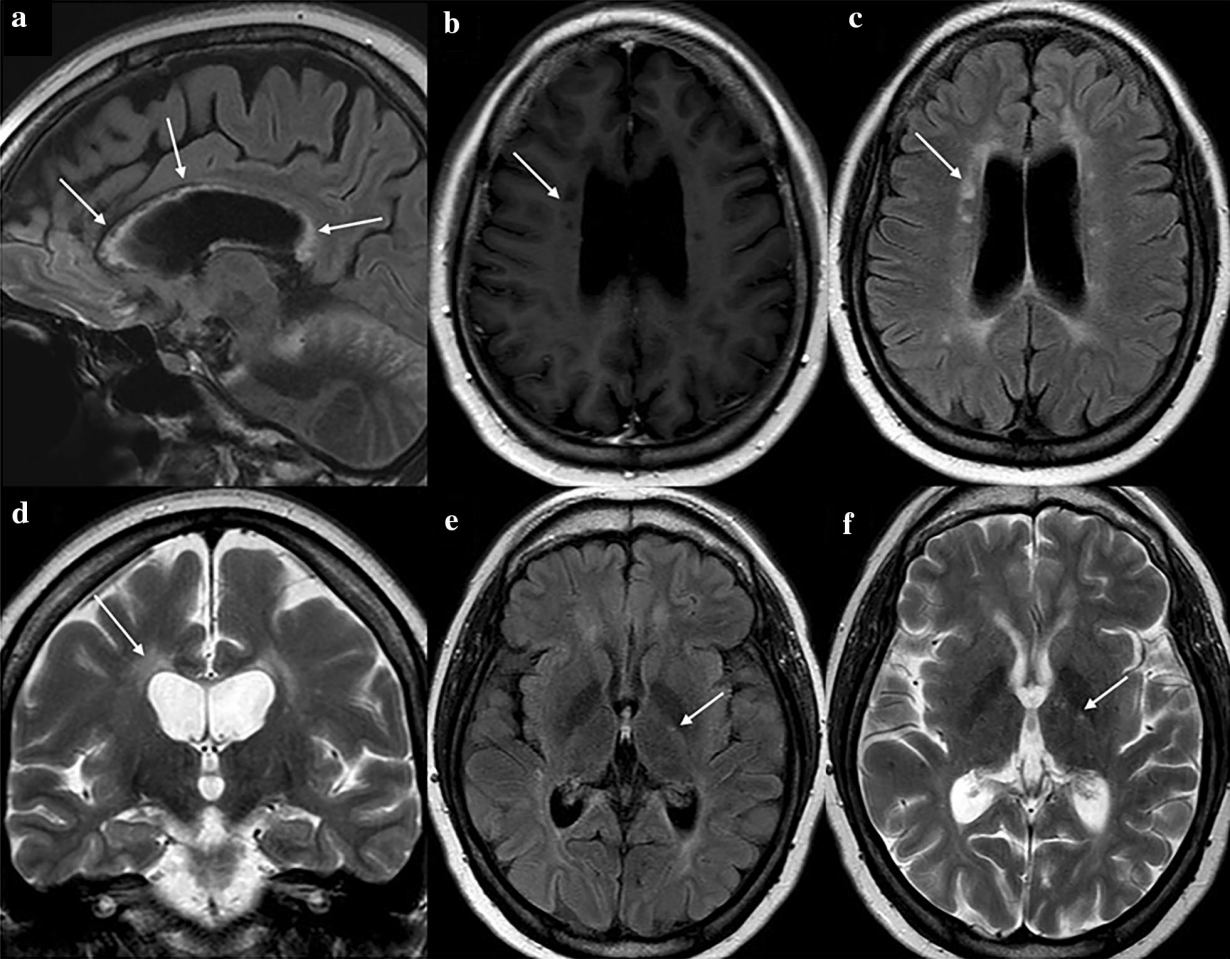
Brain magnetic resonance imaging (MRI). a Sagittal FLAIR-weighted sequence demonstrated diffuse hyperintense signal in the corpus callosum, b axial T1-weighted sequence showed multiple periventricular black holes, c, d axial FLAIR-weighted and coronal T2-weighted sequences showed periventricular lesions, e, f axial FLAIR-weighted and axial T2-weighted sequences demonstrated small lesions with hyperintense signal in the internal capsule
